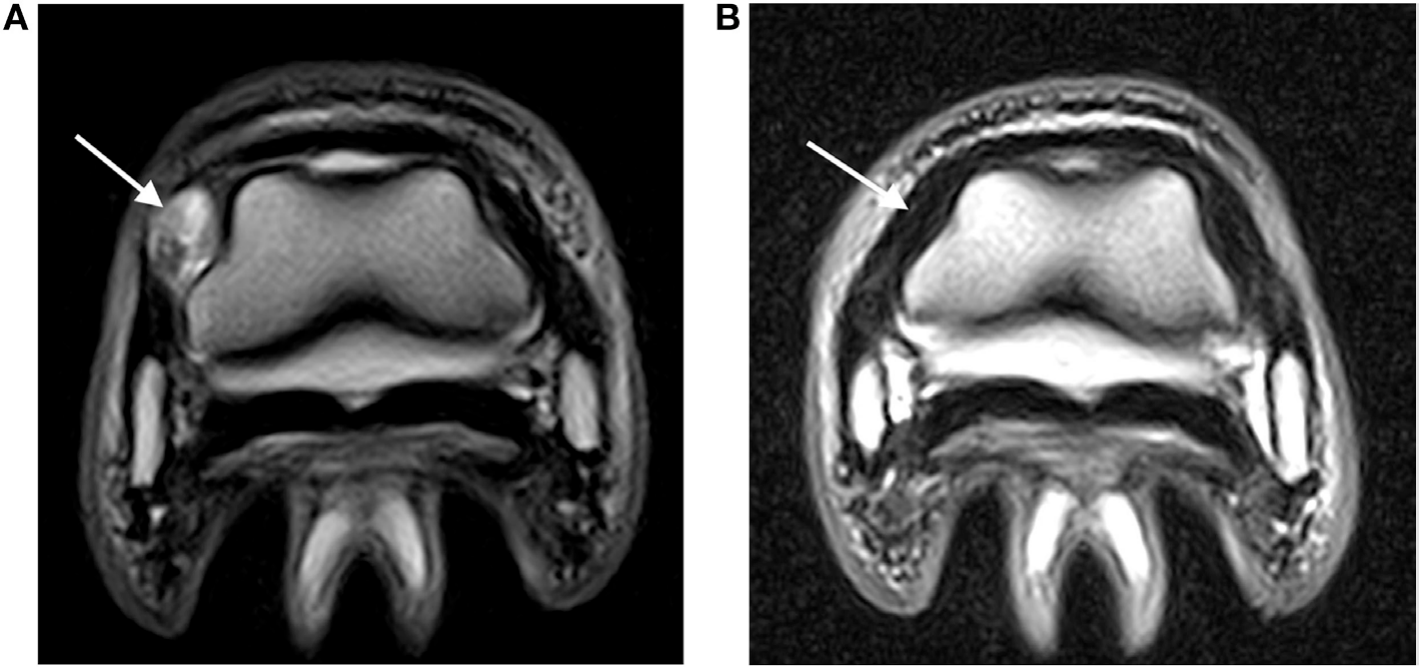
(A) Abnormal hyperintense signal (arrow) in a DIJCL prior to injection (T2-weighted fast spin echo sequence; medial is to the left). (B) Recheck MRI examination demonstrating normal signal intensity (arrow) in the same DIJCL 6 months after injection (T2-weighted fast spin echo sequence; medial is to the left).
